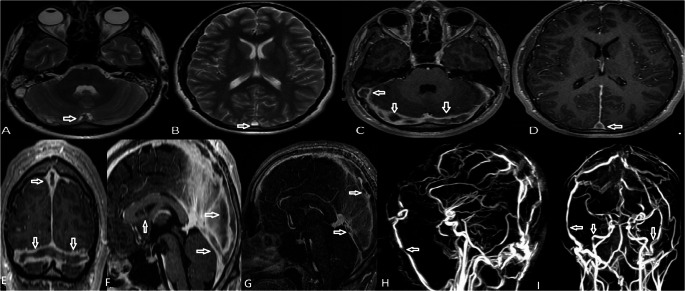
(A, B) Axial T2-weighted images demonstrate loss of the normal flow void in bilateral sigmoid sinuses, transverse sinuses, and superior sagittal sinus, consistent with thrombosis. (C, D) Postcontrast 3D T1-weighted axial images show a lack of enhancement in the same venous structures, confirming extensive thrombosis involving the bilateral sigmoid sinuses, transverse sinuses, and superior sagittal sinus. (E-G) Postcontrast 3D T1-weighted axial and sagittal images demonstrate the absence of normal enhancement in the bilateral sigmoid sinuses, transverse sinuses, sinus rectus, and superior sagittal sinus, consistent with extensive cerebral venous sinus thrombosis. (H, I) MR venography MIP images demonstrate extensive cerebral venous sinus thrombosis. Due to the absence of flow in the dural sinuses caused by thrombosis, the venous system is not visualized, and unintended visualization of the arterial system is observed
